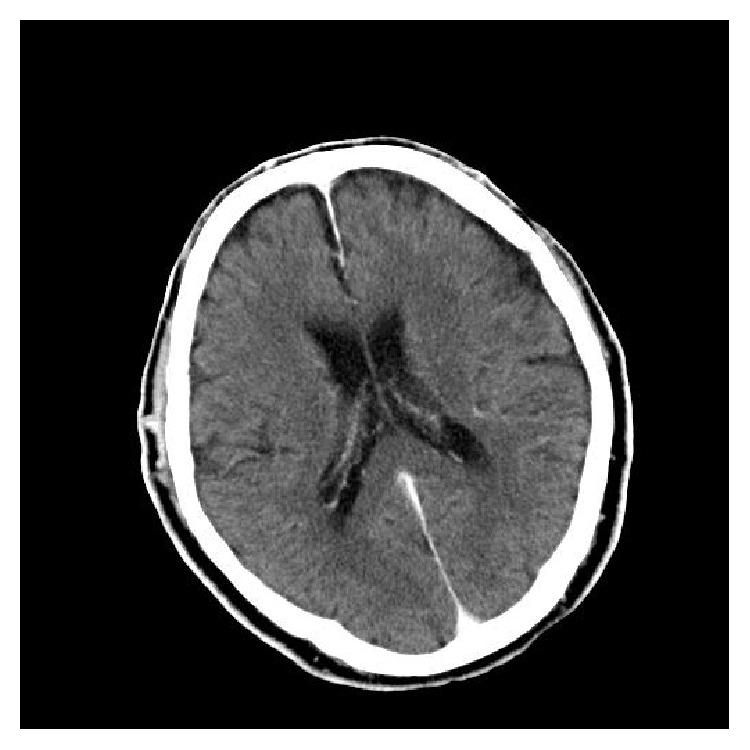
CT of the brain with intravenous contrast on the first day of admission showing no intracranial pathology, no hydrocephalus, and no brain abscess formation.
